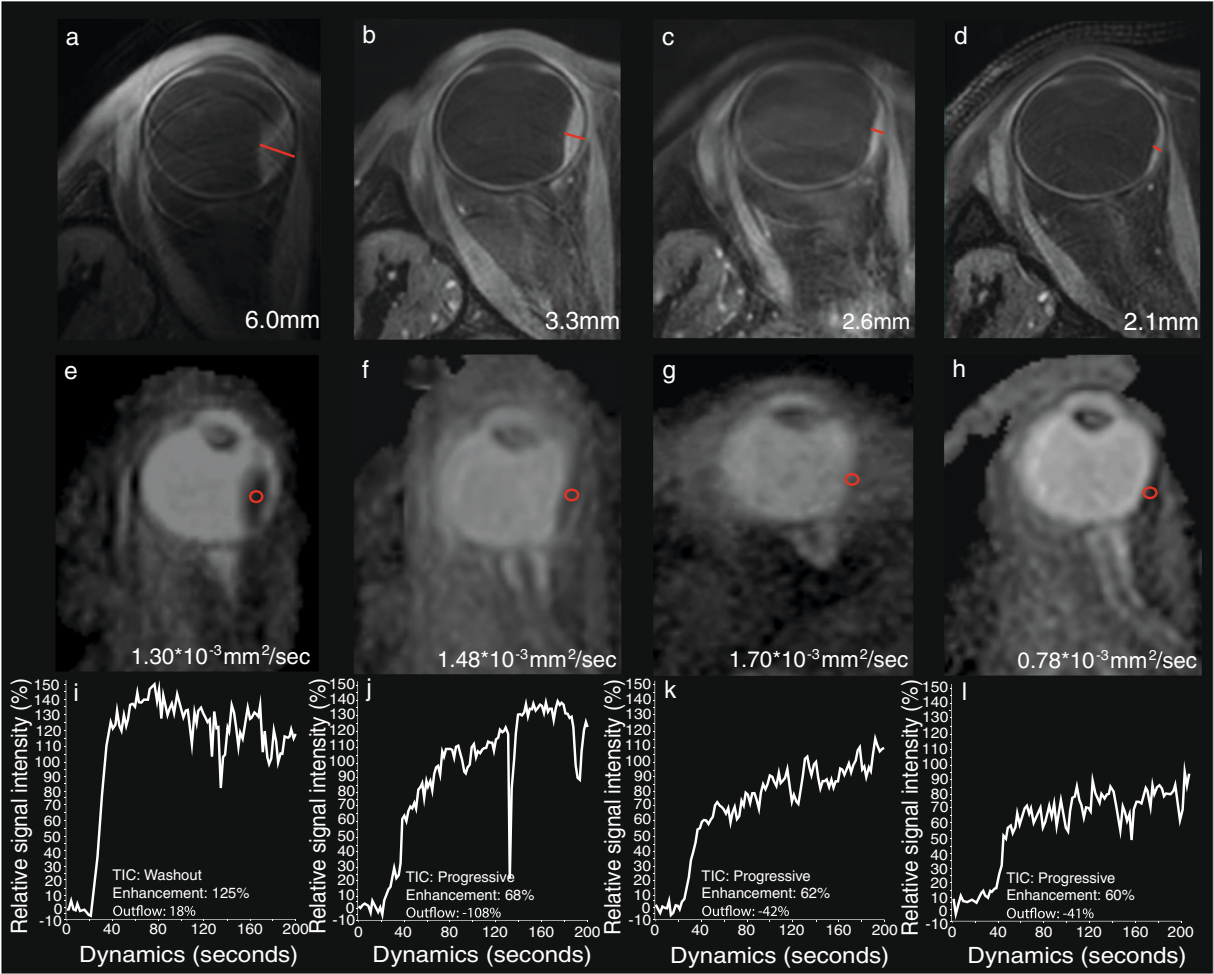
MRI of a UM of the right eye (a, e, i) before, and (b, f, j) 3, (c, g, k) 7 and (d, h, l) 13 months after ruthenium plaque brachytherapy. Favourable evolution of the UM was observed in terms of size (red lines in a–d), ADC value and TIC at dynamic contrast-enhanced MR perfusion (DCE). a–d Axial oblique multi-slice (MS) turbo spin echo (TSE) contrast-enhanced T1 with fat signal suppression. Progressive decrease in size of the UM, indicated by red lines, already noticed 3 months after radiotherapy. e–h Axial oblique ADC. Progressive increase of the UM ADC value, except 13 months after radiotherapy where there is a decrease. Regions of interests used to derive the ADC values are delineated in red circles. i–l DCE TICs. Progression of the initial washout TIC profile into a plateau and progressive TIC, corresponding to a lower (more negative) outflow percentage
